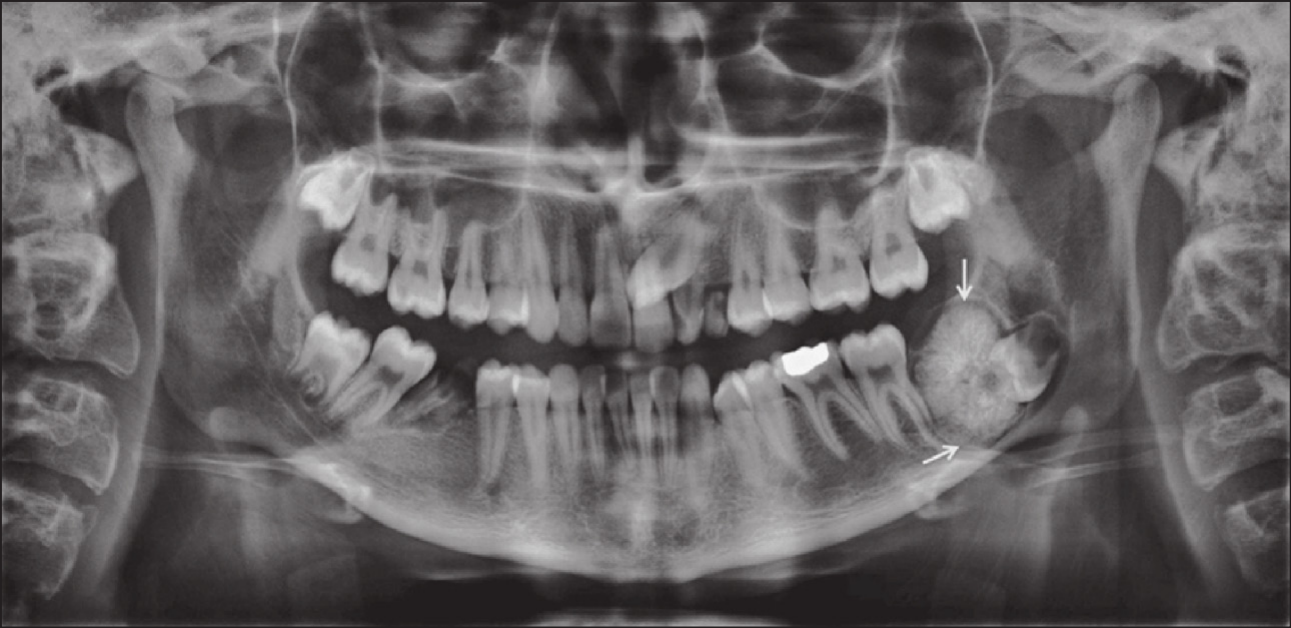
A panoramic radiograph shows a large complex odontoma associated with the impacted left mandibular third molar. Reprinted with permission from Mortazavi, Hamed & Baharvand, Maryam. (2016). Jaw lesions associated with impacted tooth: A radiographic diagnostic guide. Imaging Science in Dentistry. 46. 147. 10.5624/isd.2016.46.3.147.
